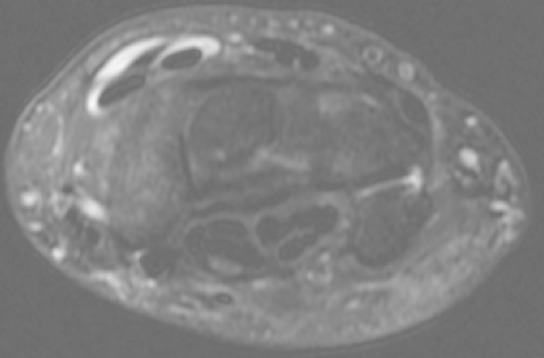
MRI axial view showing thickening and effusion peritendinous at the junction of ECRB and ECRL with extensor pollicis brevis (EPB) and abductor pollicis longus (APL)
